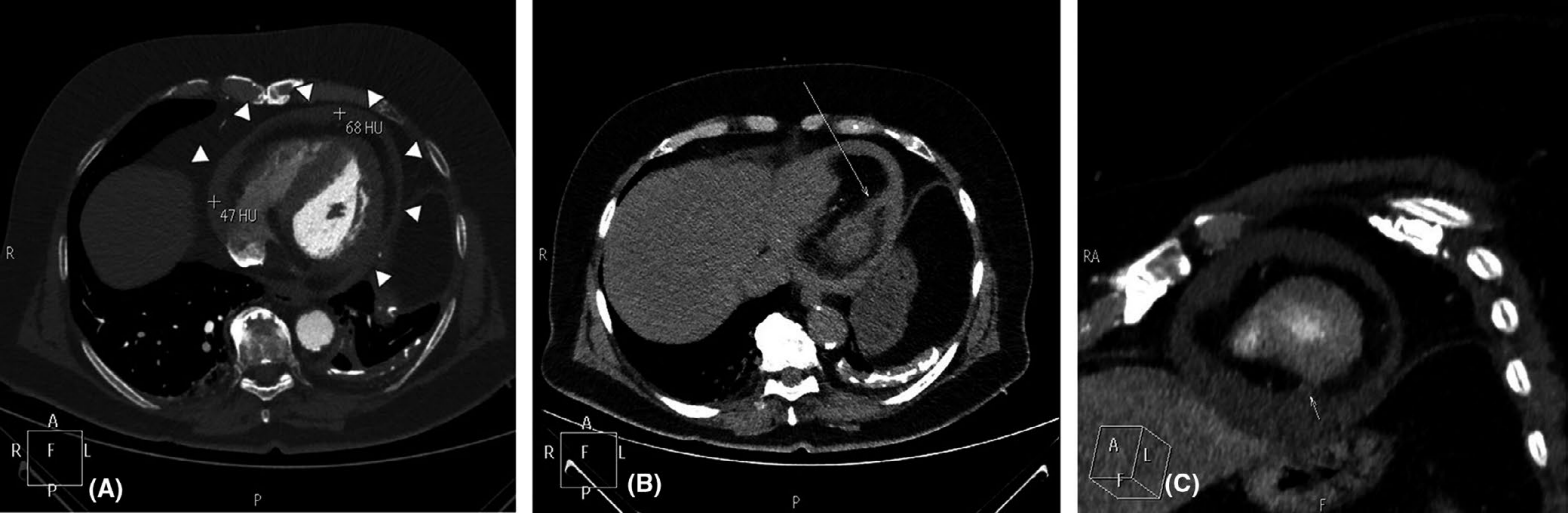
A, Axial CT imaging showing hemopericardium (white arrowheads); (B and C) Reconstructed CT images showing the site of perforation in the inferolateral wall (thin white arrows)
